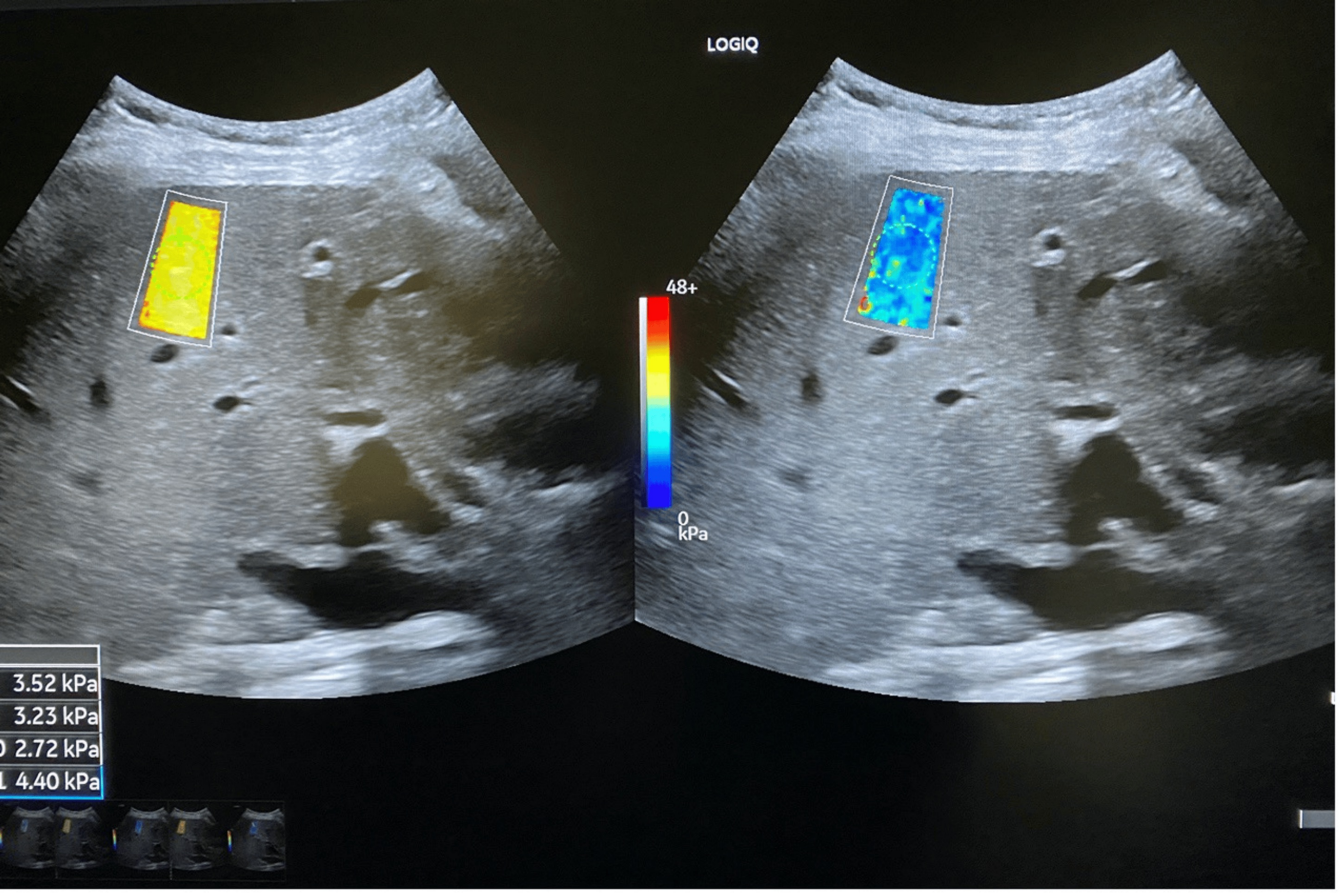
Normal 2D-shear wave elastography The ultrasound images shown are part of our team's original work and were obtained by the authors as part of this study.
